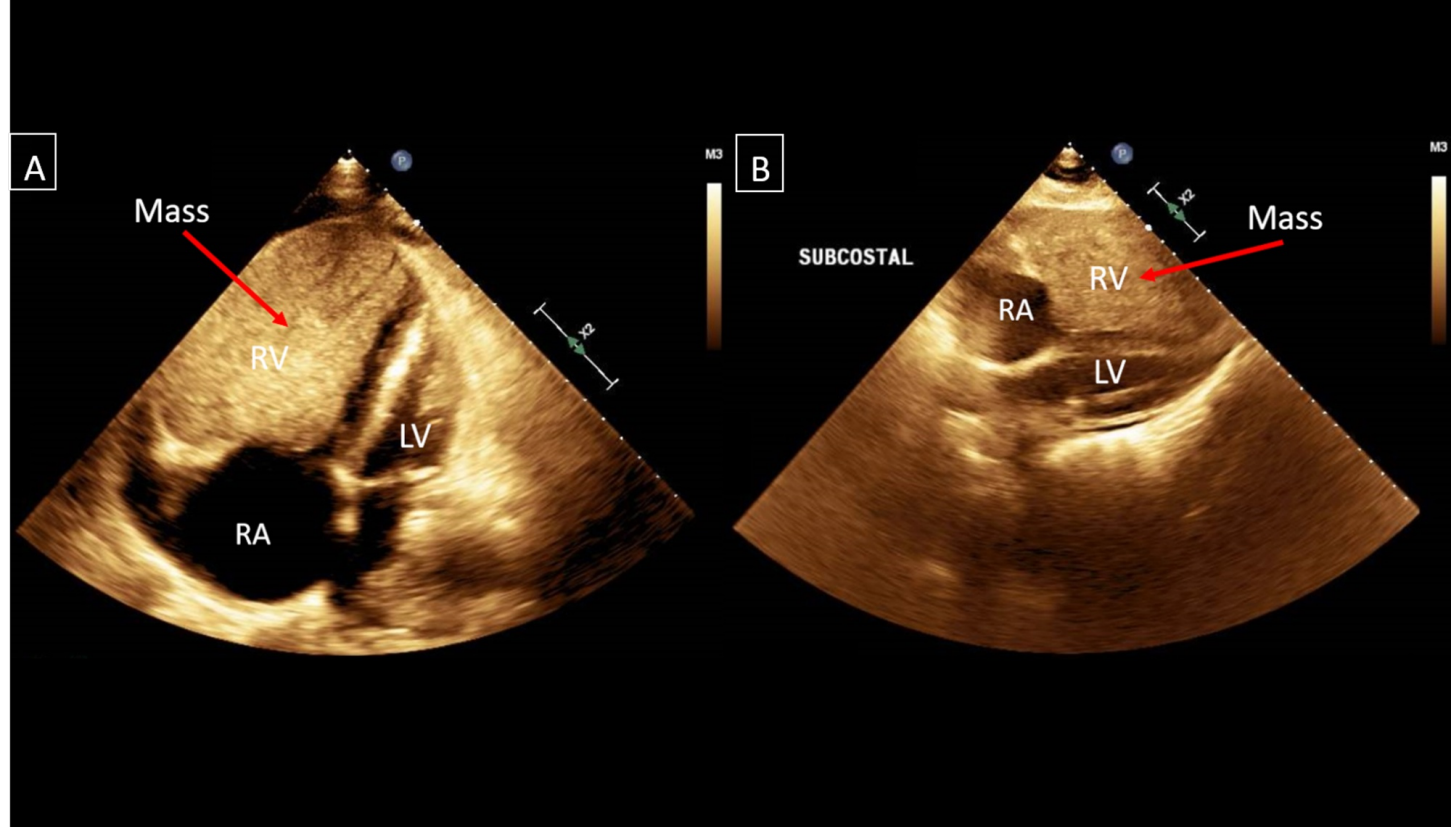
(A&B) Transthoracic echocardiogram in parasternal four-chamber view and subcostal view demonstrating a well circumscribed homogenous mass within the right ventricle measuring 8.10 X 6.54 cm and compressing the left ventricle. RA: Right Atrium; RV: Right Ventricle; LV: Left Ventricle
